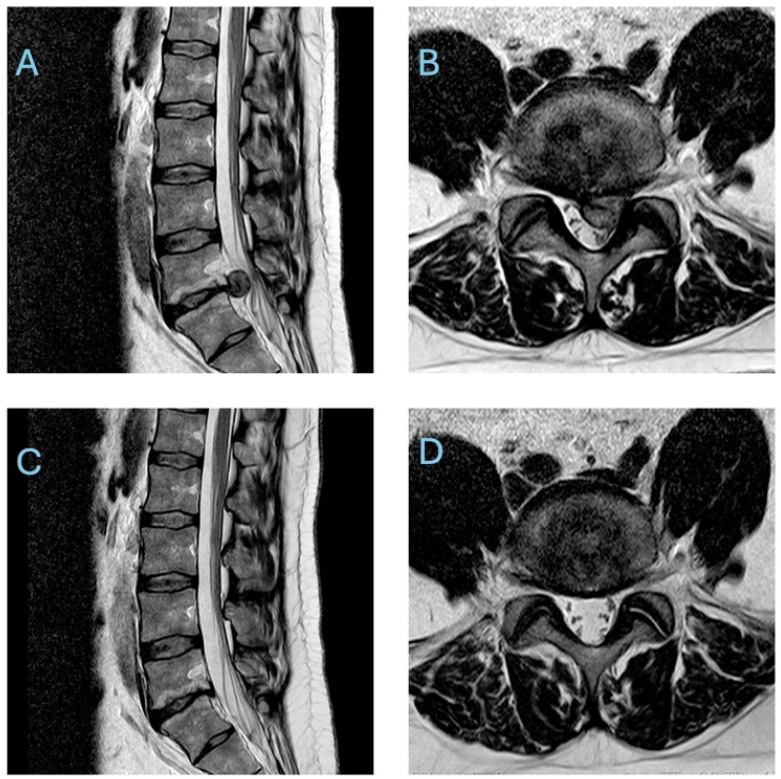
T2 weighted MRI images of a lumbar disc herniation demonstrating spontaneous resorption. The sagittal (A) and axial (B) views show a large disc extrusion compressing neural elements before treatment. After five months of conservative management, follow-up sagittal (C) and axial (D) scans of the same patient show complete disappearance of the herniated disc material, with relief of neural compression.
